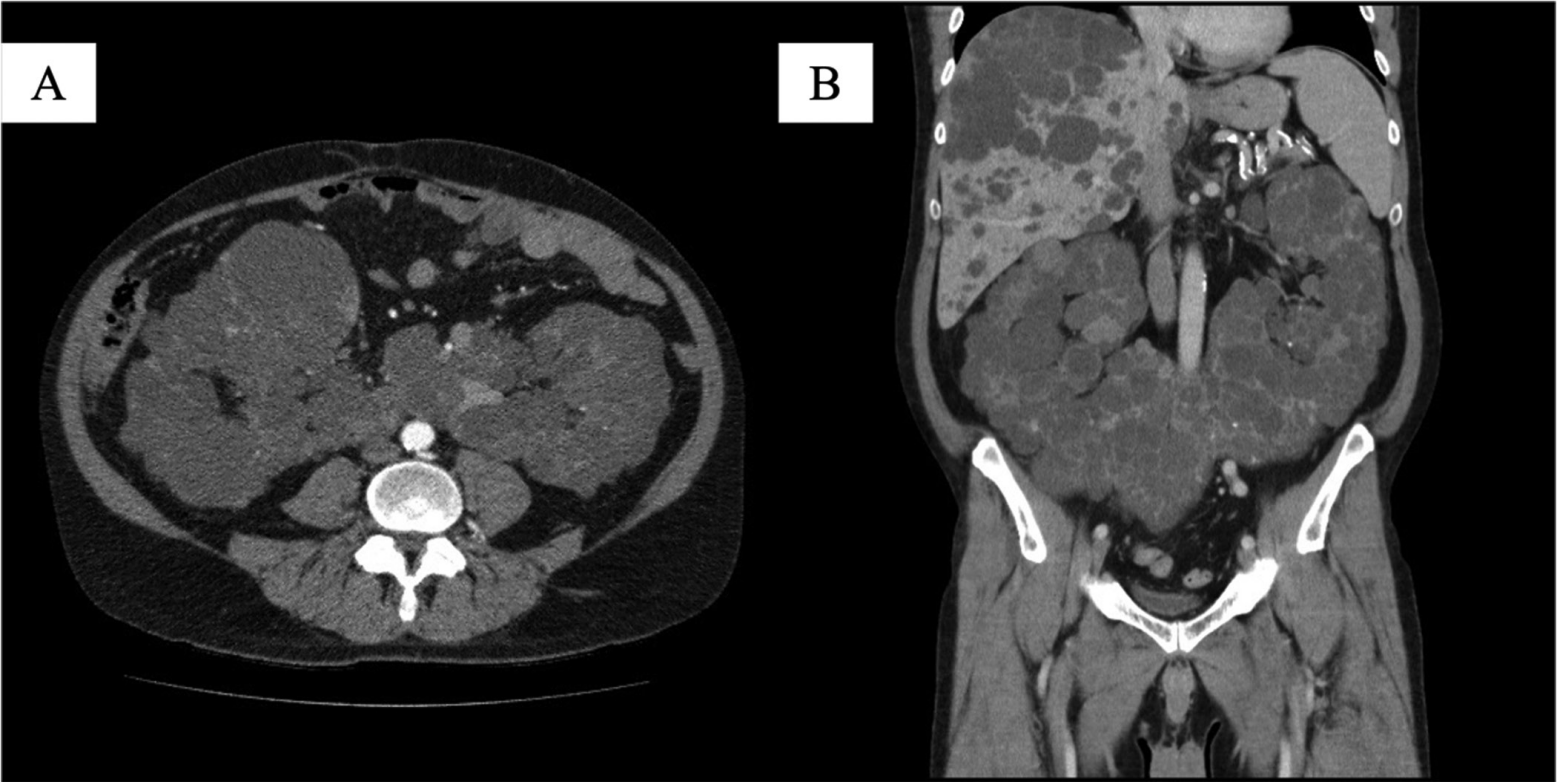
Computed tomography (CT) showing renal fusion and massive cysts. A: Axial section; B: Coronal section.
